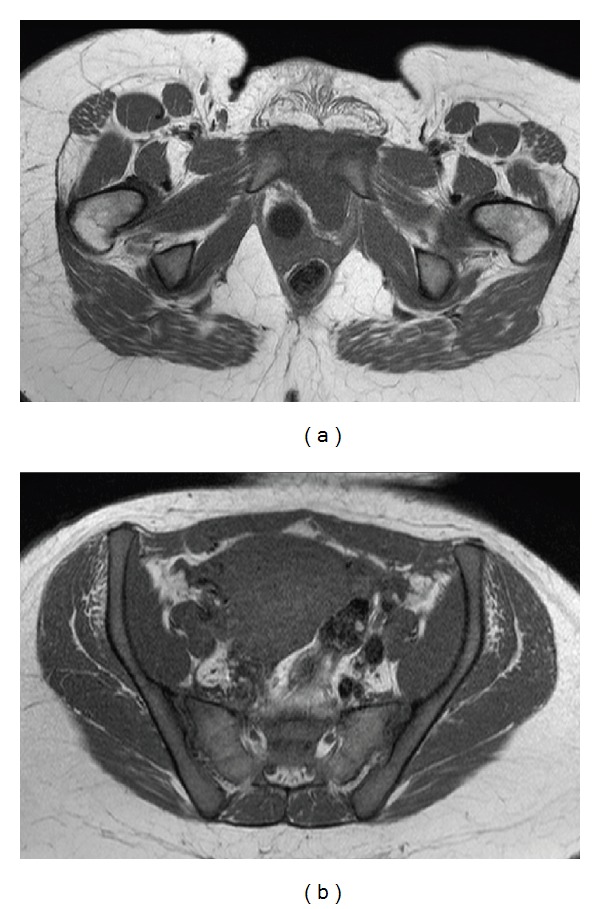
Axial T1-weighted images without contrast are shown in (a) and (b). (a) shows the MRI that confirms the pubic symphysis separation. No separation of the iliosacral joints and no pelvic fracture can be identified in (b).
